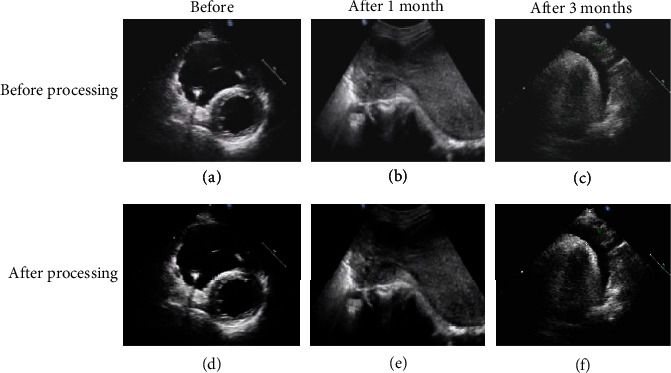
Ultrasonic images of experimental group at each time period.
